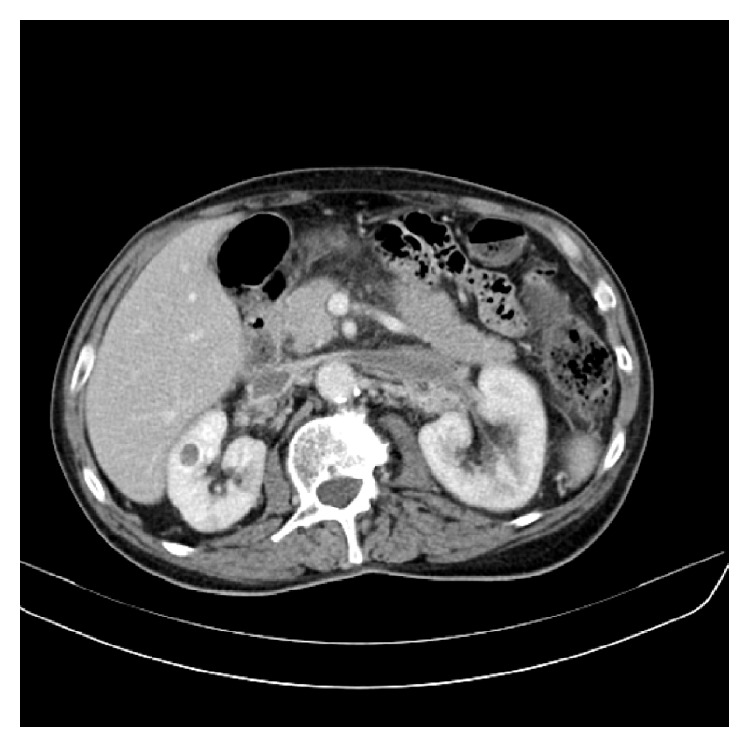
CT scan cross section: thrombosis of the left and right renal veins and of the portal vein.
